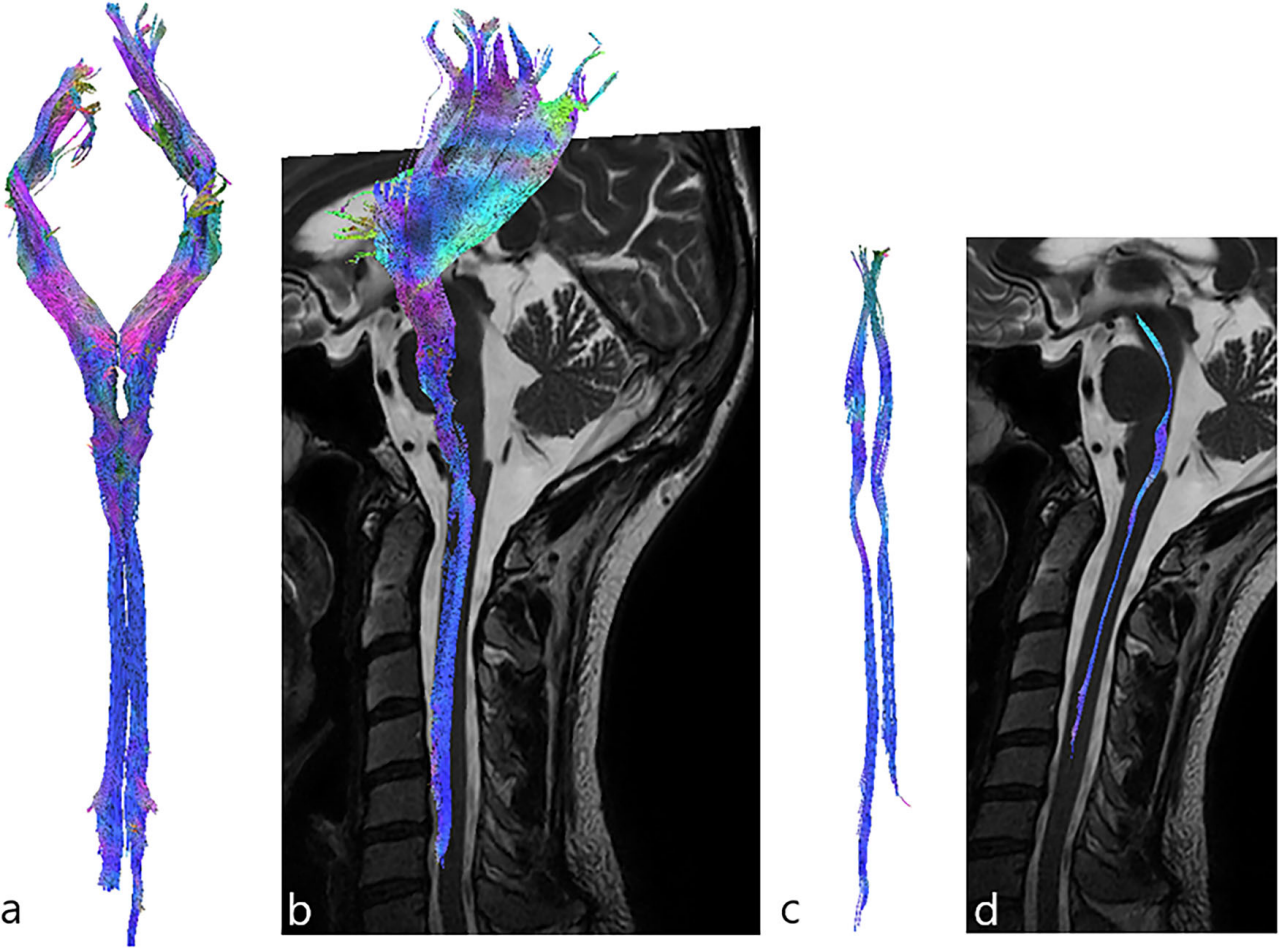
3D-tractography rendering of motor pathways. a Coronal view of the corticospinal tract. b Sagittal view of the corticospinal tract, merged on sagittal T2-weighted images. c Coronal view of the rubrospinal tract. d Sagittal view of the rubrospinal tract merged on sagittal T2-weighted images
